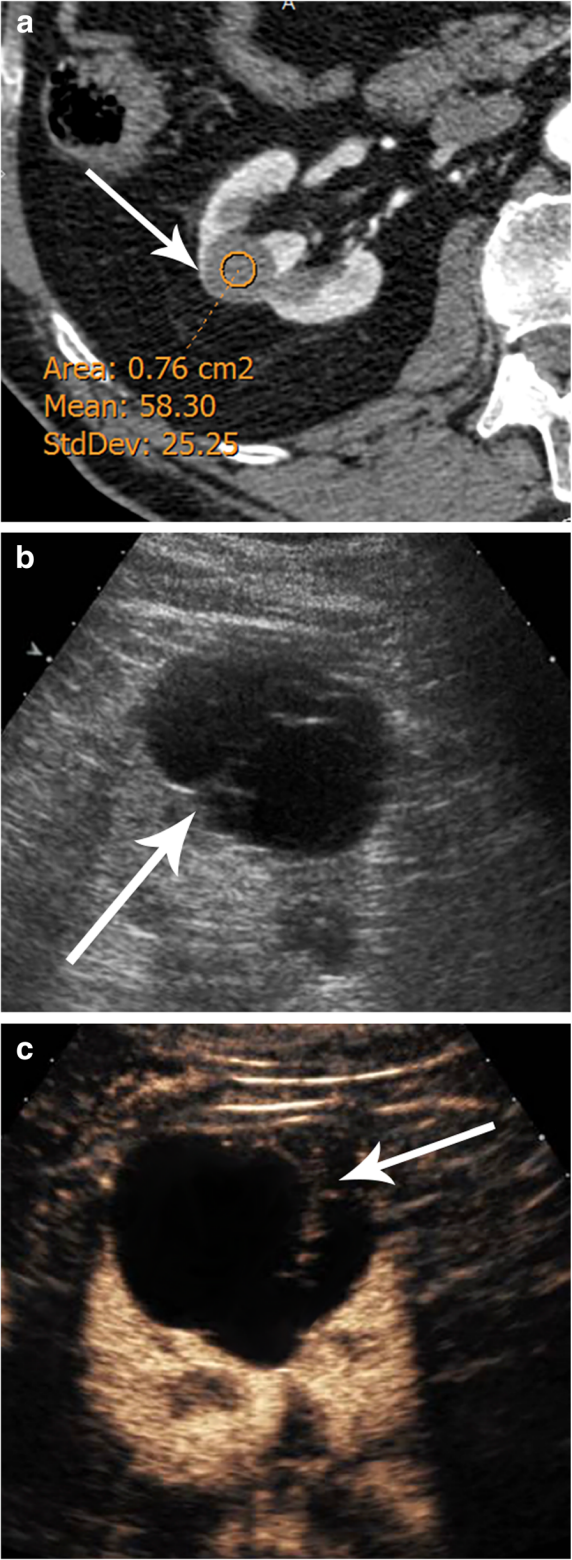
Indeterminate renal mass by CT in the right kidney. a Axial CT in the arterial phase depicts a renal tumor with 58 HU consistent with the indeterminate lesion (arrow). b Unenhanced and (c) contrast-enhanced ultrasound (US) of the same renal tumor showing the presence of a cyst with thin septa with no contrast uptake corresponding to a Bosniak II lesion (arrow): follow-up was not required
